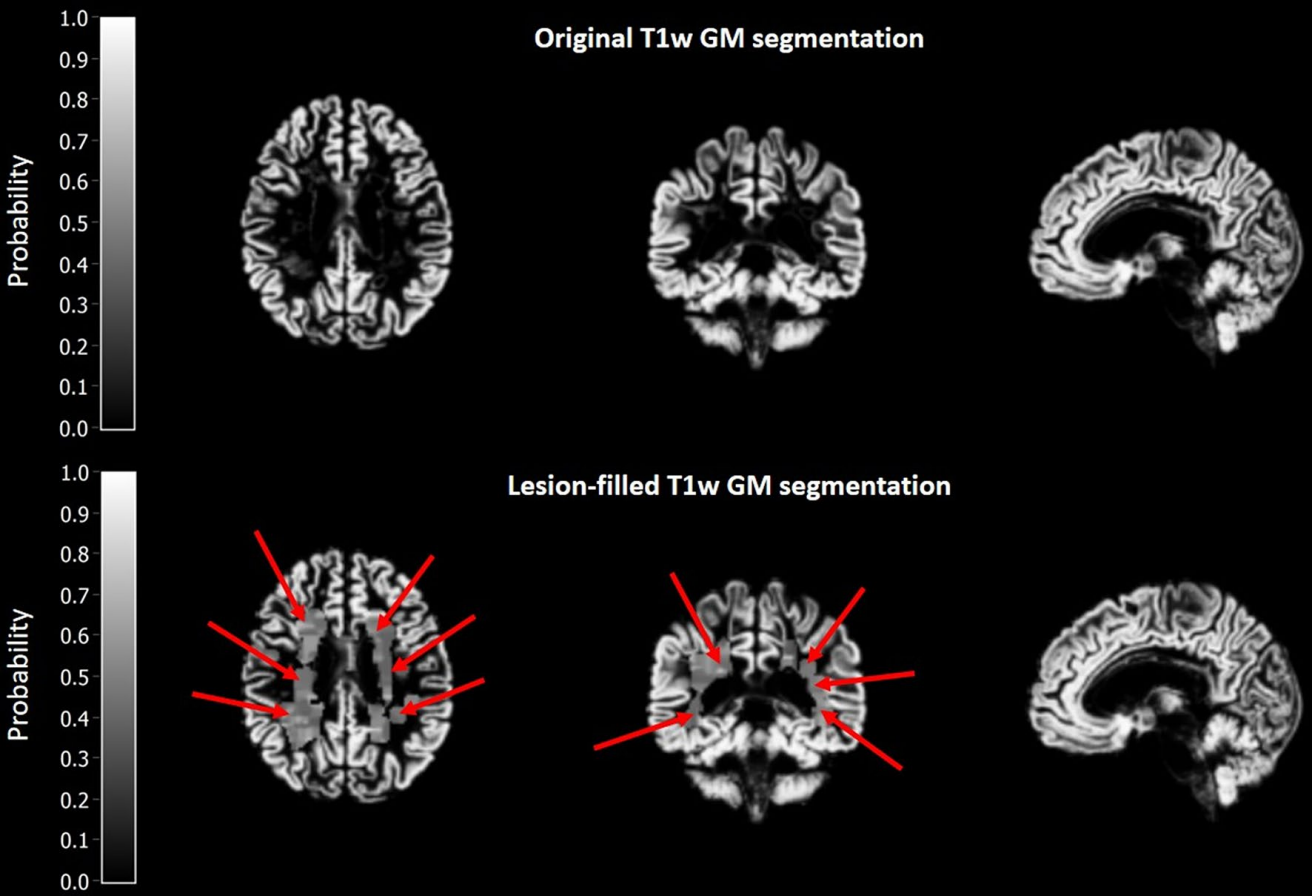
GM segmentation of both original T1w and lesion-filled T1w MRI
